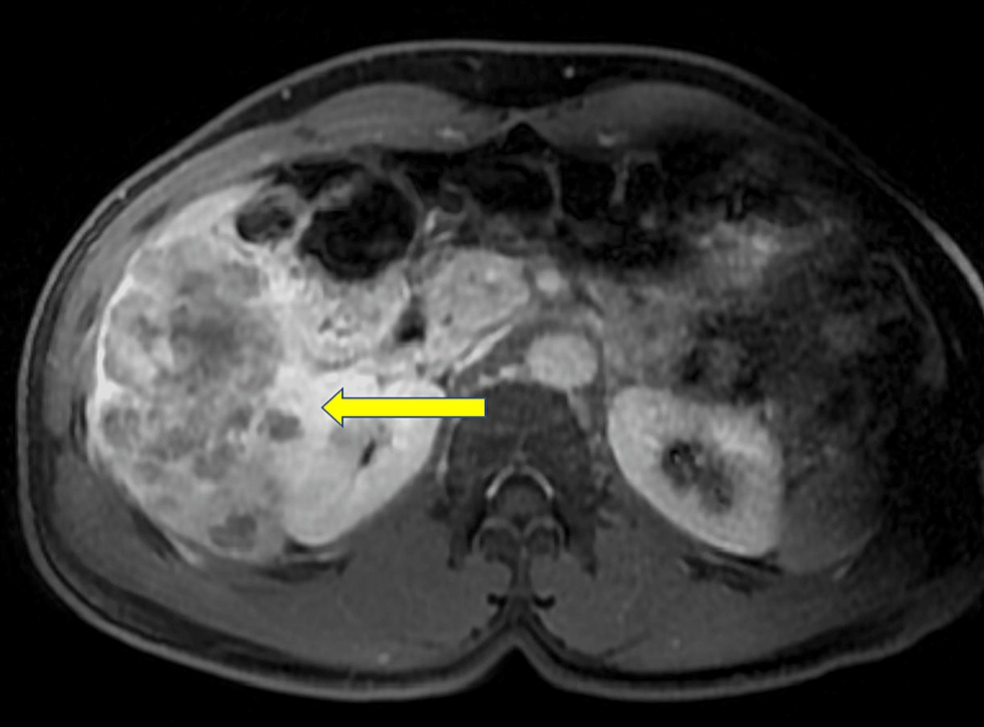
An axial MRI image with contrast showing a 9 cm conglomerate mass made up of multiple targetoid masses in the right liver lobe (arrow).
